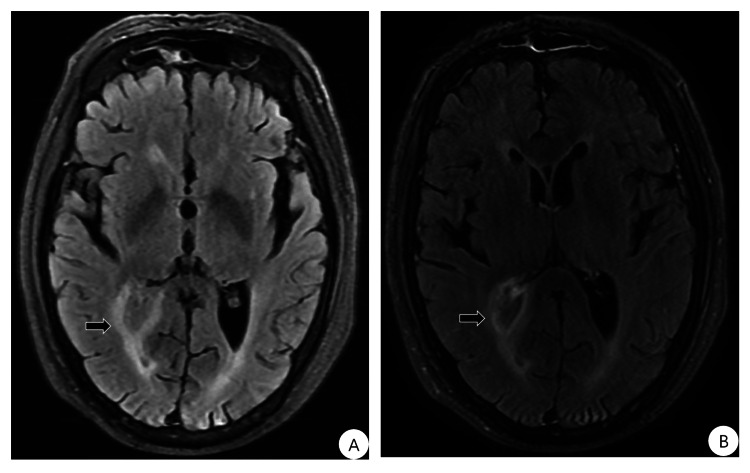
Brain MRI showed increased signal around the posterior horn of the right lateral ventricle in the right parietal lobe in both T2/FLAIR and contrast-enhanced images. T2/FLAIR (A) and enhanced FLAIR (B).
